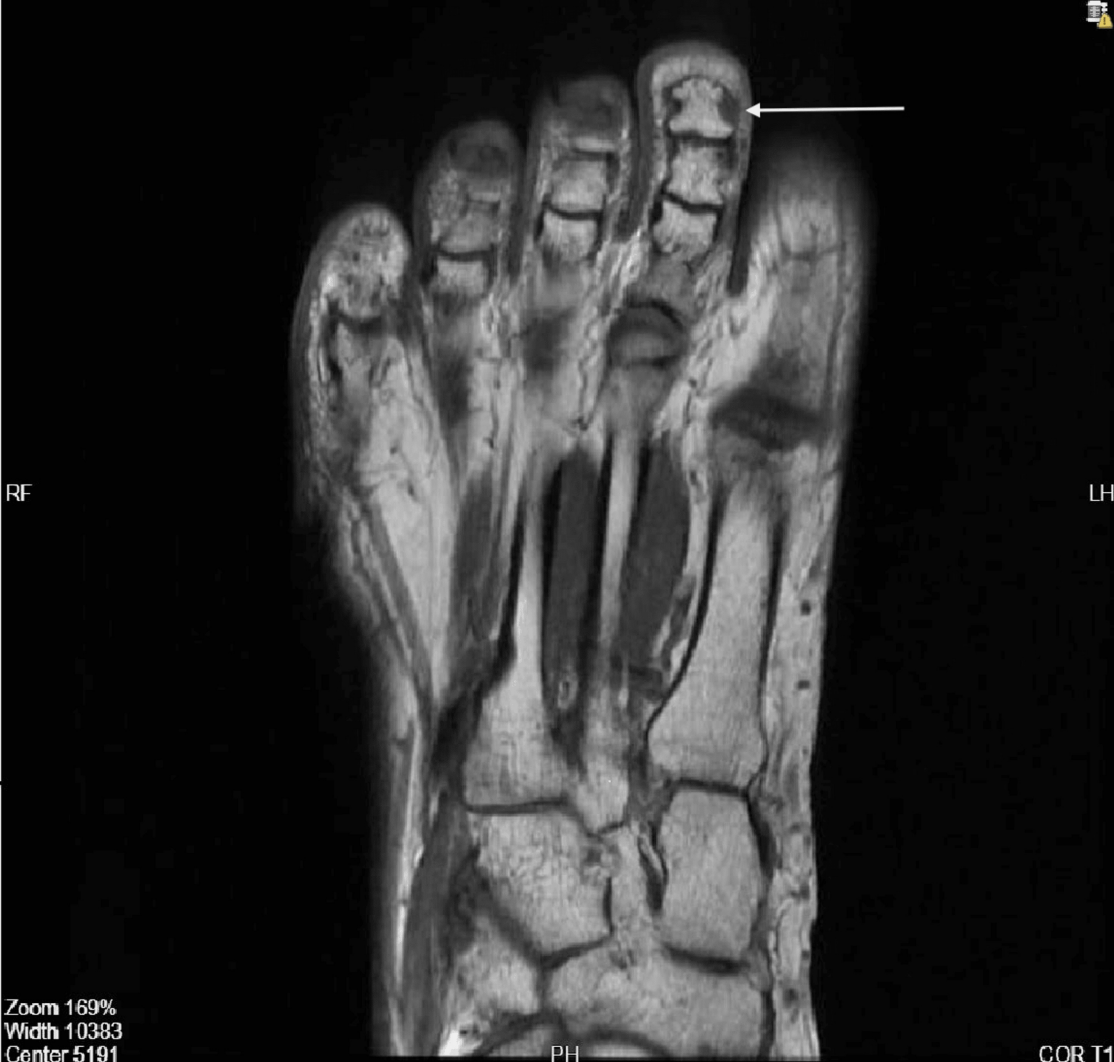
MRI of the second toe of the right foot (2023). There is no evidence of a subcutaneous soft tissue or webspace mass in the second toe of the right foot, as labeled by the arrow.
